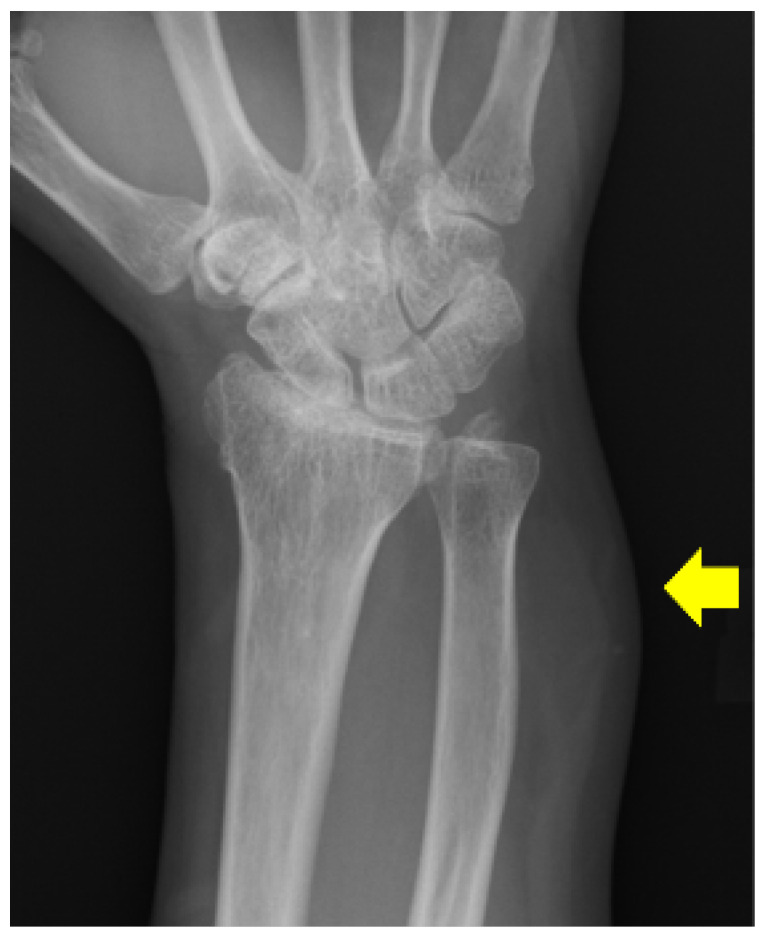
The patient was a 54-year-old woman who noticed a mass on the ulnar side of her left wrist, proximal to the ulnar head, ~6 months before her initial visit to our hospital. This mass gradually increased in size, prompting her to consult a local clinic, where she was referred to our institution for further evaluation. There was no redness or swelling of the overlying skin, and neither pain nor tenderness was present. Tinel’s sign was negative, and no limitation of the normal range of motion was observed. The patient had a history of two distal radius fractures of the left wrist caused by falls that had occurred four and three years prior. The first injury had been treated surgically, whereas the second was managed conservatively. On initial radiographs, a fat-dense lesion was observed on the lateral side of the ulna (yellow arrow), without evidence of calcification or bony deformity. Mild narrowing of the wrist joint space was noted, attributable to the previous distal radius fractures.
